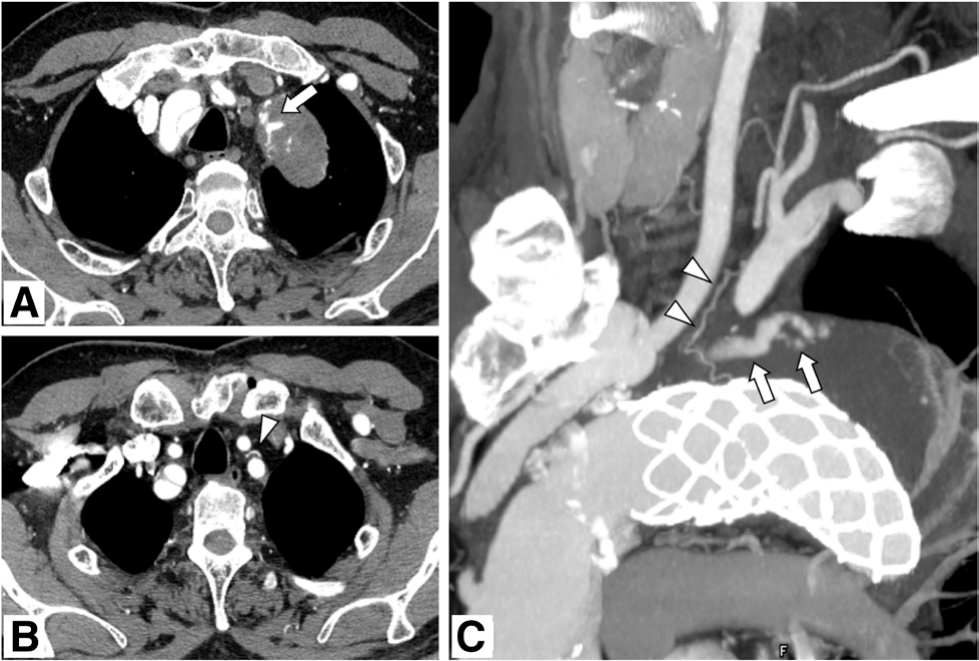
Pre-treatment contrast-enhanced CT. (A, B) Contrast enhancement within the aneurysm sac suggestive of an endoleak (arrow) and a small mediastinal branch originating from the proximal portion of the left subclavian artery, suspected to be the responsible vessel (arrowhead), is observed. (C) Slab MIP image in the left anterior oblique view shows the endoleak within the aneurysm sac (arrows) and the small mediastinal branch (arrowheads). CT: computed tomography; MIP: maximum intensity projection
